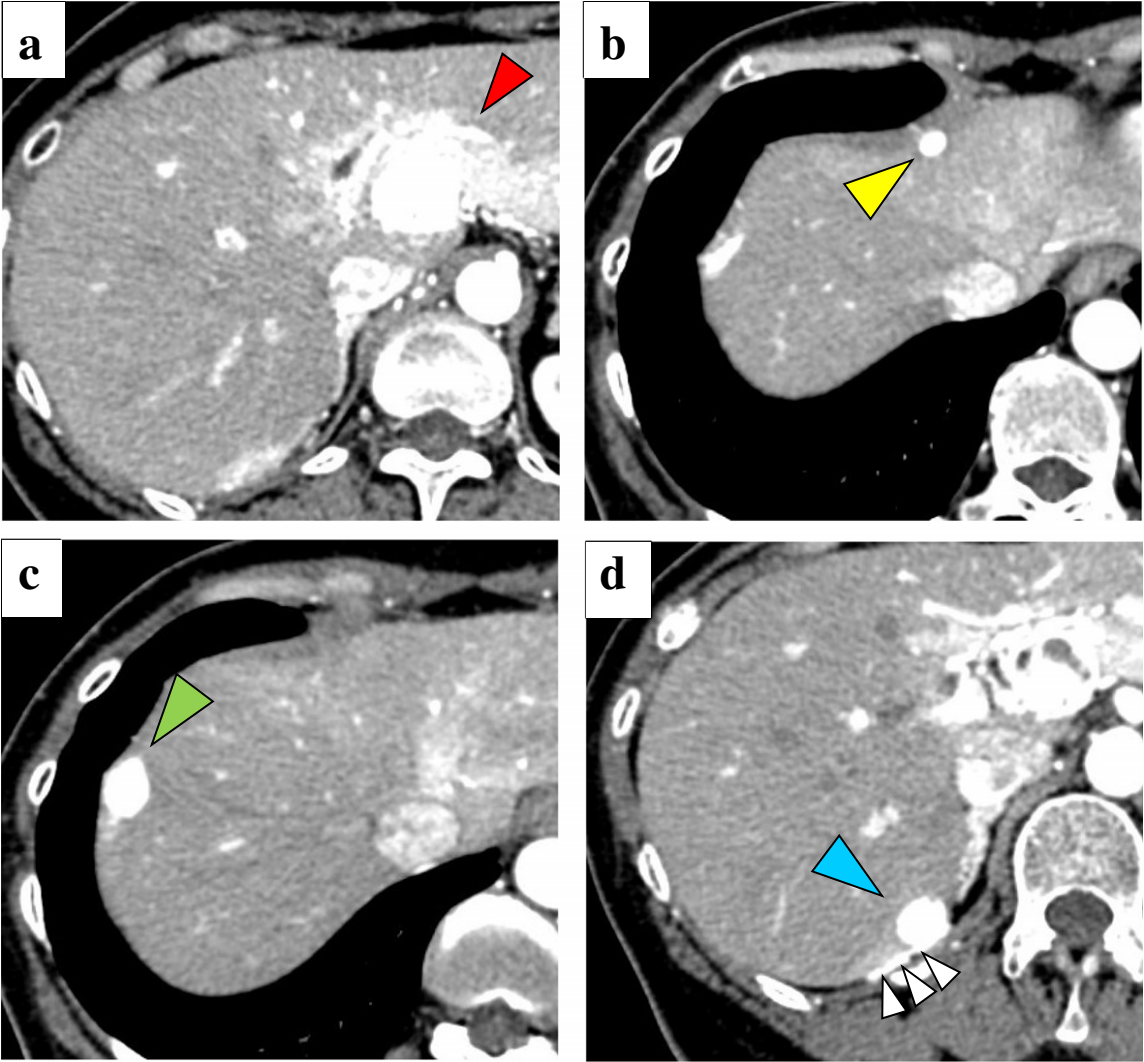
Enhanced abdominal computed tomography showing a hypervascular tumor measuring 32-mm diameter (a, *red arrow*) in contact with the lateral liver segment. A 7-mm tumor in segment 4 (b, *yellow arrow*), 15-mm tumor in segment 8 (c, *green arrow*), and 17-mm tumor in segment 7 (d, *blue arrow*) are also seen. All tumors are positioned on the liver periphery. Moreover, the tumor in segment 7 is continuous with the diaphragm and has the same high density of contrast medium (*white arrows*)
